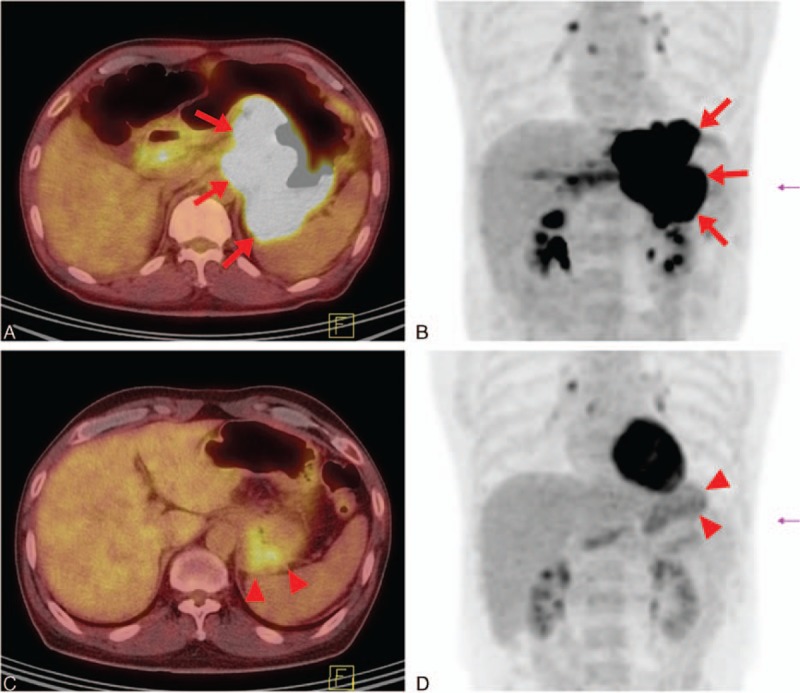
A 53-year-old man with gastric diffuse large B-cell lymphoma. In baseline positron emission tomography/computed tomography (PET/CT) transaxial (A) and PET maximum intensity projection (MIP) (B) images, intense F-18-fluoro-2-deoxyglucose (FDG) uptake was seen along the gastric wall from cardia to body, mainly along the lesser curvature side (maximum standardized uptake value 28.2) (arrows). In interim PET/CT transaxial (C) and PET MIP (D) images after 3 cycles of first-line chemotherapy, previously noted FDG uptake in the stomach showed markedly decreased intensity and extent (arrowheads). Remaining FDG uptake could not be differentiated from physiological gastric activity. Reader A interpreted this finding as Deauville score 4 and reader B interpreted it as score 1. After completion of first-line chemotherapy, the patient remains in clinical remission without additional therapy (overall survival 76.8 months). CT = computed tomography, FDG = F-18-fluoro-2-deoxyglucose, MIP = maximum intensity projection, PET = positron emission tomography.
